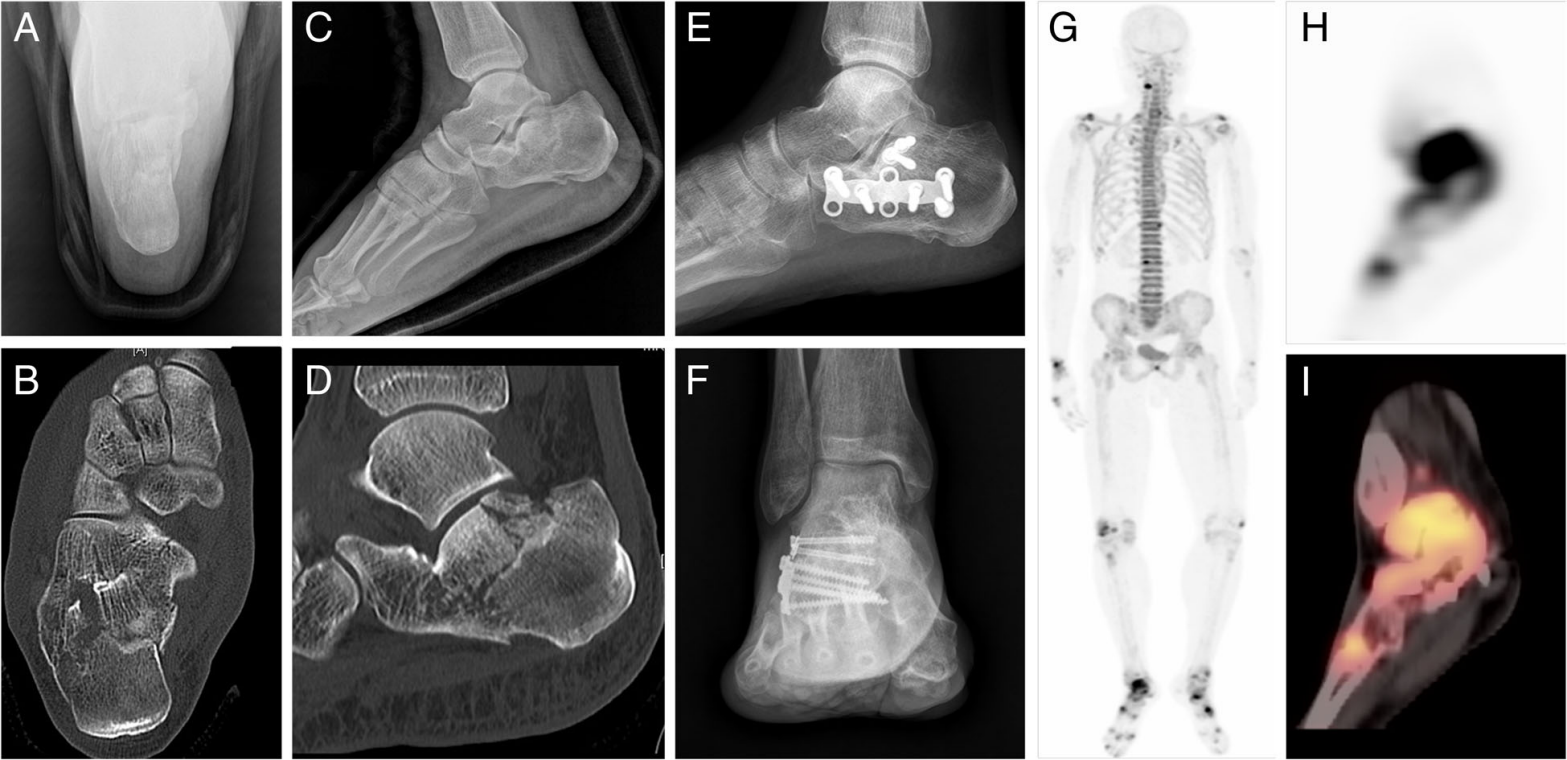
Radiologic and ^18^F-sodium fluoride (18F-NaF) positron emission tomography-computed tomography (PET/CT) images of a 53-year-old male patient who had a right calcaneus fracture and underwent operation with plate-screw fixation. a-d Initial images of plain radiographs and computed tomography (CT); a calcaneal anterior-posterior view, b calcaneal CT axial view, c ankle lateral view, d calcaneal CT lateral view. Note that the fracture did not involve the talocrural (tibiotalar) joint. e, f Plain radiographs performed at 9 months after injury presents plate-screw fixation, but does not give any information about talocrural joint pathology and the degree of ankylosis in the ankle. g-i 18F-NaF PET/CT shows hot uptake around the talocrural joint away from the initial fracture and plate-screw fixation area
